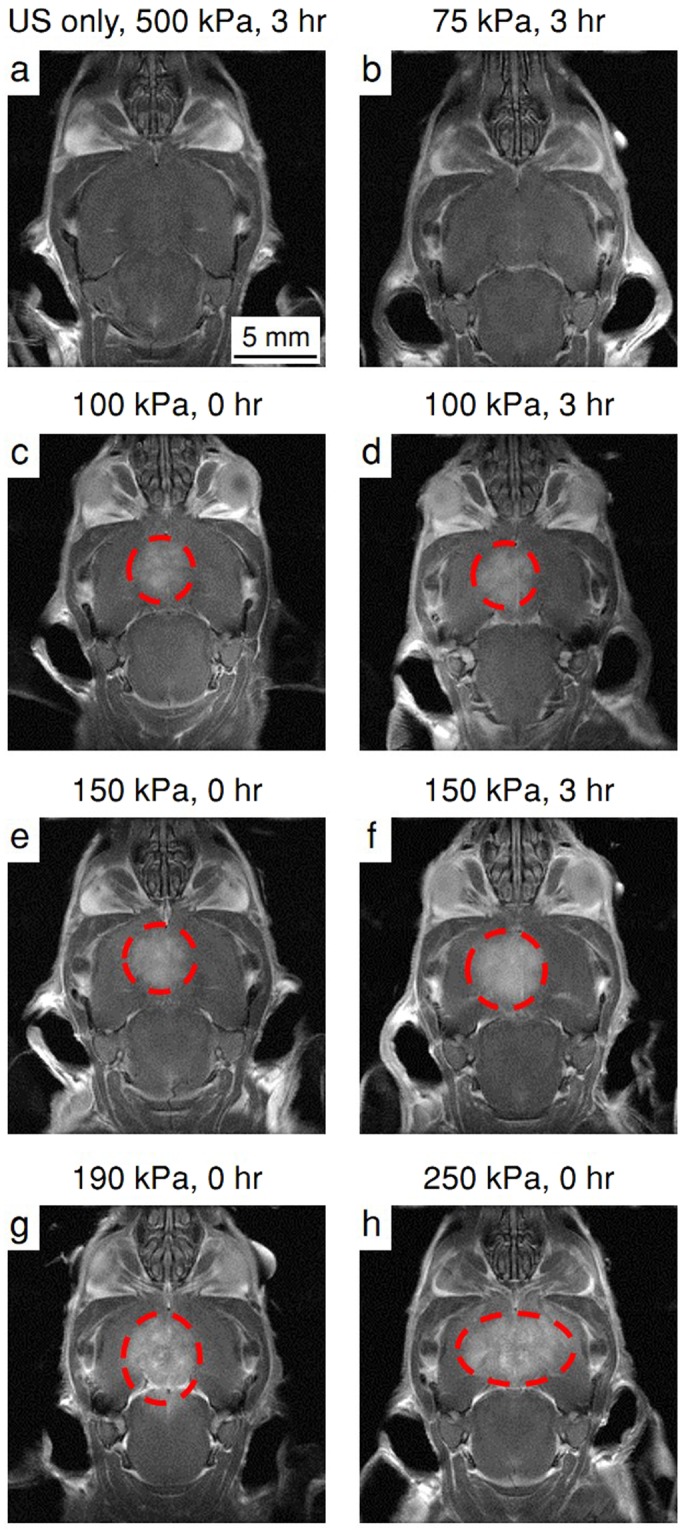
Coronal T1-weighted MR images. The contrast enhancement area is indicated by the red dashed circles. (a) US (ultrasound)-only with 500 kPa peak negative pressure (PNP), 3 hours post treatment. (b) ultrasound + MBs with 75 kPa PNP, 3 hours post treatment. (c,d) Ultrasound + MBs with 100 kPa PNP, at 0 and 3 hours post treatment respectively. (e,f) Ultrasound + MBs with 150 kPa PNP, at 0 and 3 hours post treatment respectively. (g) Ultrasound + MBs with 190 kPa PNP, at 0 hours post treatment. (h) Ultrasound + MBs with 250 kPa PNP, at 0 hours post treatment.
